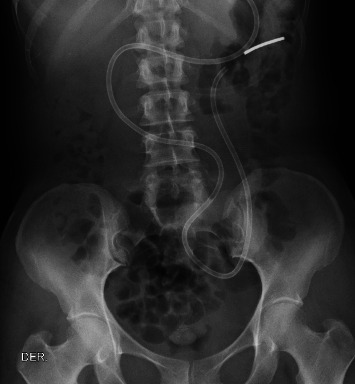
Supine abdominal radiograph showing the course of a nasoenteric tube reaching into the small bowel. Multiple mildly dilated small bowel loops with air-fluid levels are seen, consistent with a proximal obstruction pattern.
